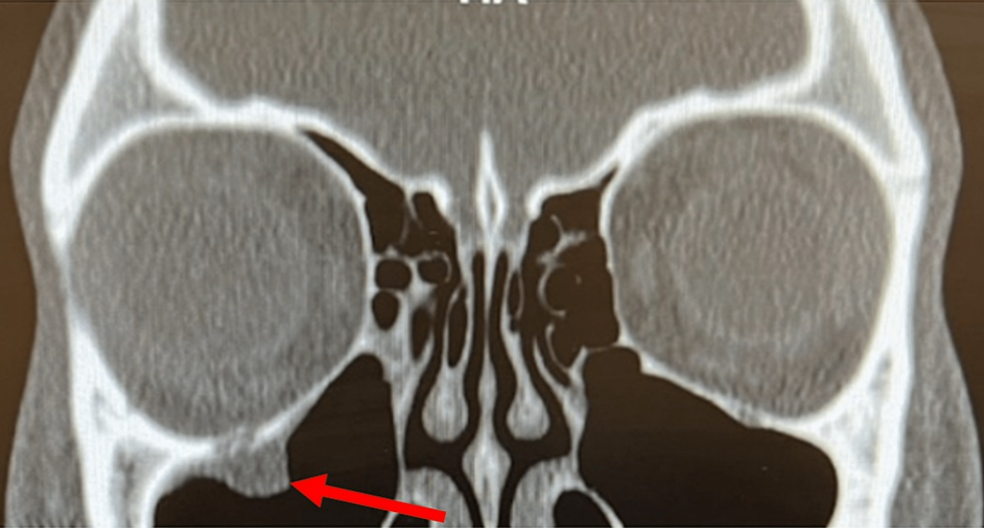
A coronal view of the non-contrast CT (soft tissue window) showing a left-sided orbital floor trapdoor fracture and associated orbital soft tissue entrapment in the fracture (red arrow).
